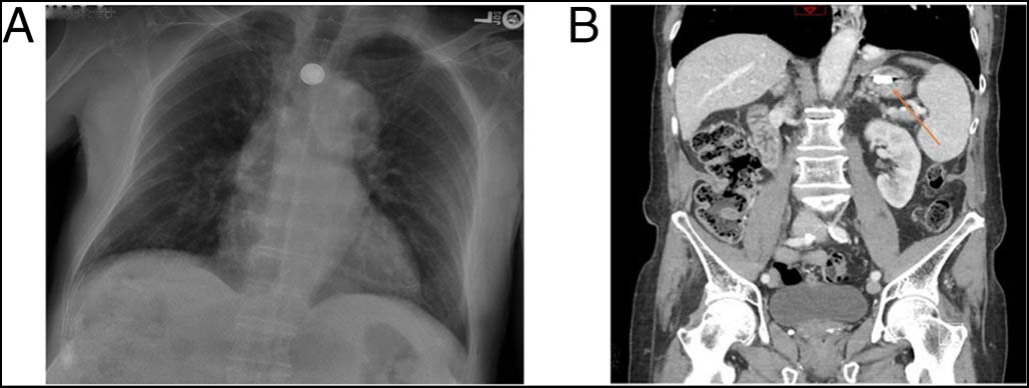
(A) Initial portable chest radiograph and (B) abdominal computed tomography revealed a 20-mm round, disc-like radiopaque foreign body within the middle 1/3 of the esophagus.
